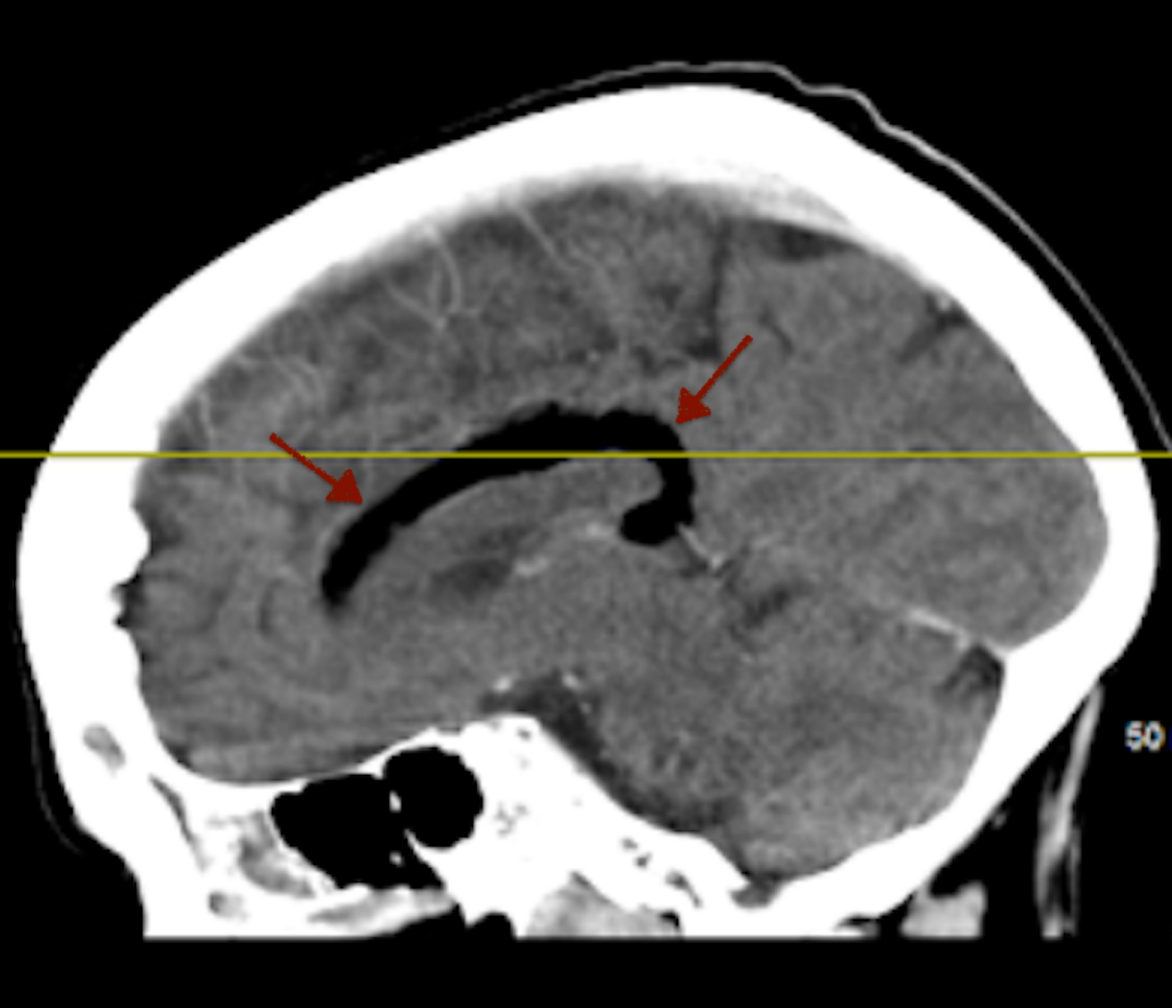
Sagittal CT of the head Sagittal CT of the head demonstrates a tubular area of hypodense fat attenuation (red arrows) along the superior callosal margin that curves around the splenium of the corpus callosum.
